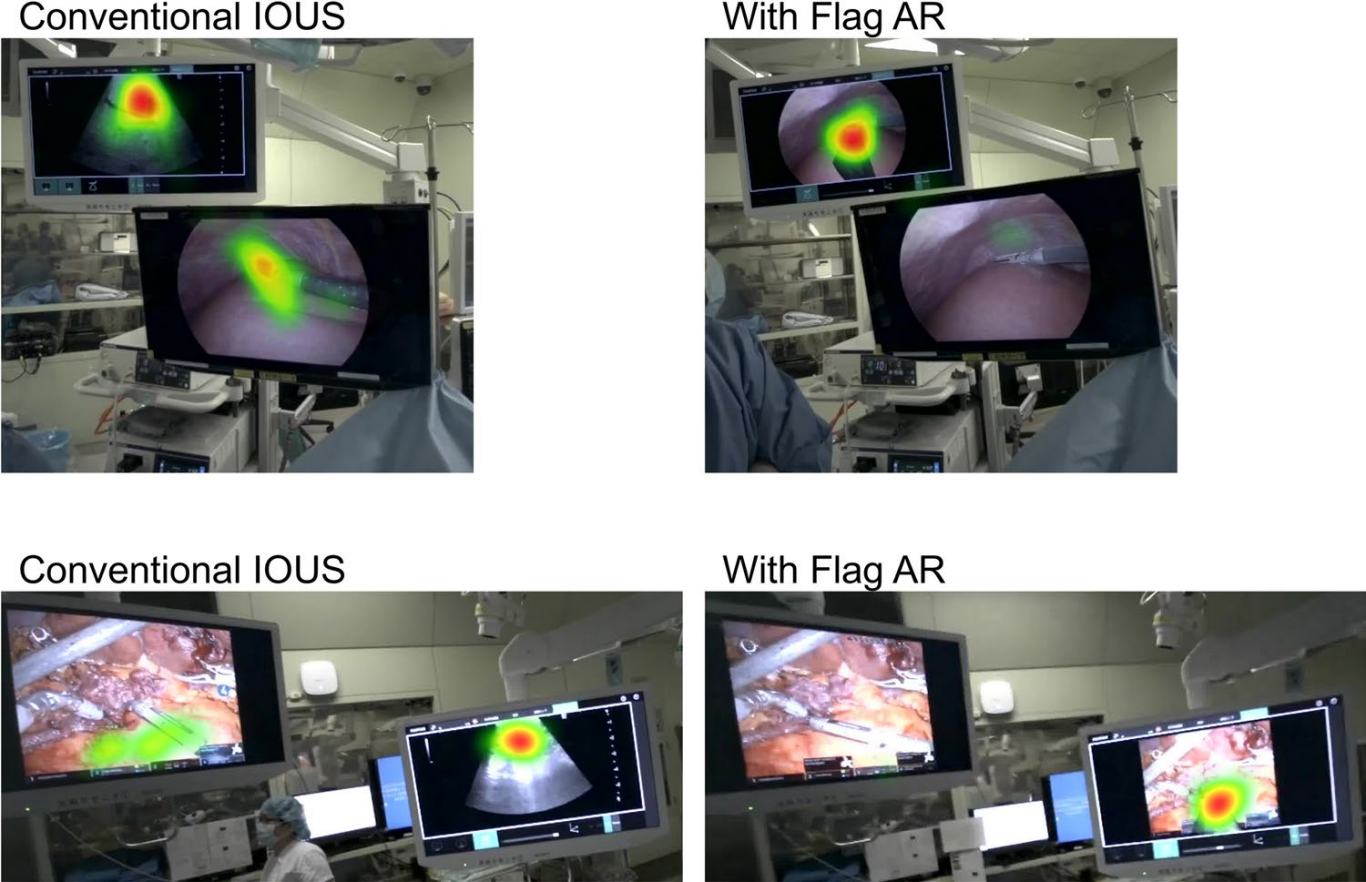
Surgeon gaze pattern analysis during target identification. Left panels (Conventional IOUS): Surgeons are required to monitor both the endoscopic display (lower monitor) and separate ultrasound monitor (upper monitor), resulting in dispersed visual attention across multiple displays as shown by the heat map overlay. Right panels (With Flag AR): The AR superimposition system enables surgeons to focus visual attention predominantly on the endoscopic monitor, as the ultrasound image is directly overlaid onto the laparoscopic field of view. Heat map visualization clearly demonstrates concentrated gaze patterns on a single display when using Flag AR, corresponding to the quantitative finding of 97.9% single-monitor focus time compared to 73.8% with conventional IOUS
